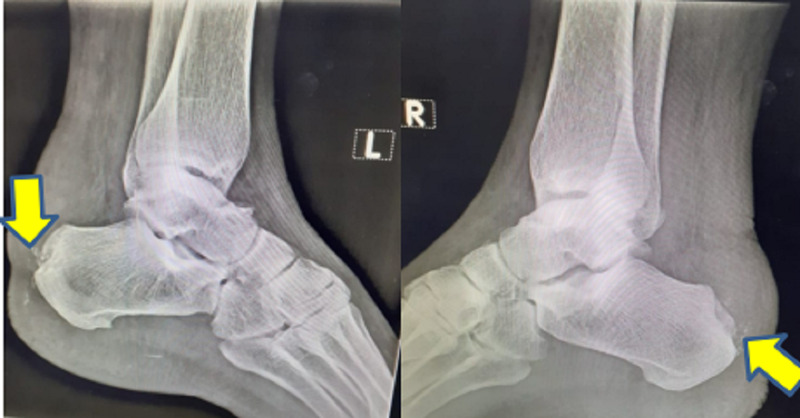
Postoperative lateral ankle radiograph following surgical excision
